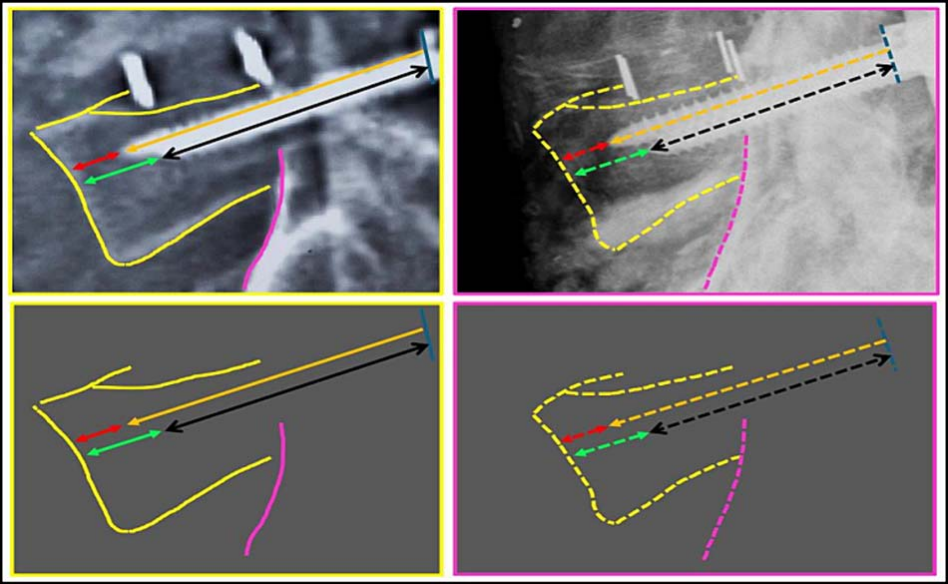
Contour of the L5 vertebral body. Upper panels, comparison between the antemortem CT images (left) and postmortem lateral X-ray (right). Lower panels, respective concordant templates.
